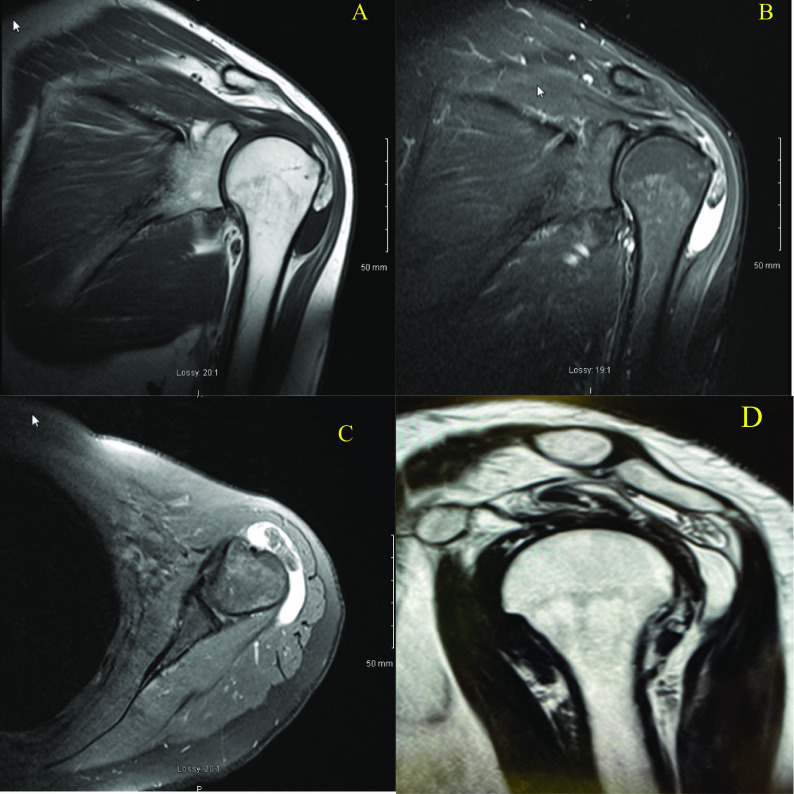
Extra-articular lipoma arborescens. (A) T1 coronal MRI (B) T2 coronal MRI (C) T2 axial MRI and (D) T1 sagittal MRI showing: fat-containing, lobulated synovial mass (2.5 × 1.0 × 0.5 cm) in the subacromial-subdeltoid bursa with fat signal intensity on all sequences.
